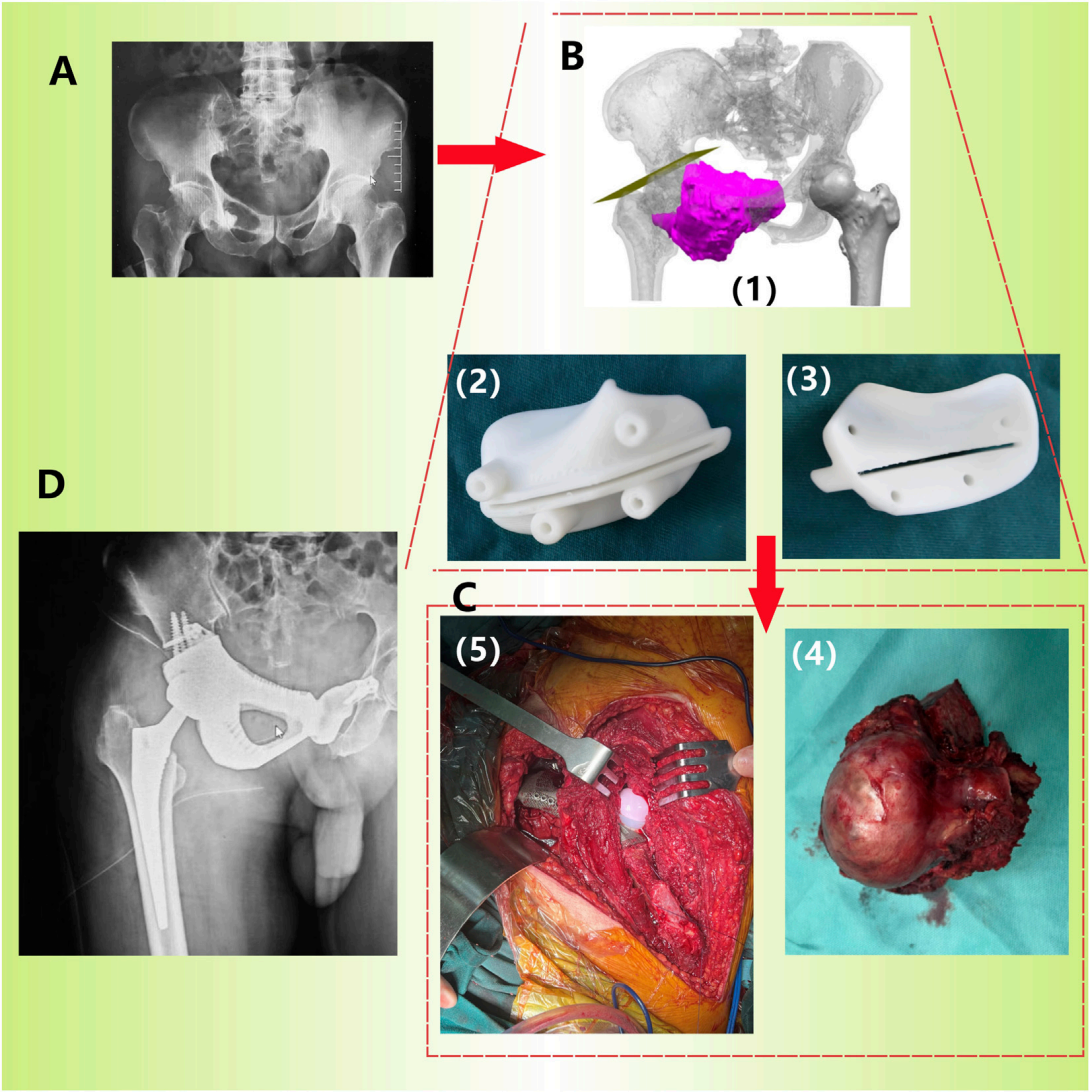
Procedure of prosthetic implantation surgery (Patient 2). (A) Preoperative pelvic X-ray images show lesions in the right pelvis; (B) Preoperative 3D planning: determination of osteotomy plane (1) and printing of osteotomy guide plate (2/3: different angles); (C) Accurate resection of tumors (4) and implantation of prostheses (5) using guide plates during surgery; (D) X-ray image taken 1 month after surgery.
